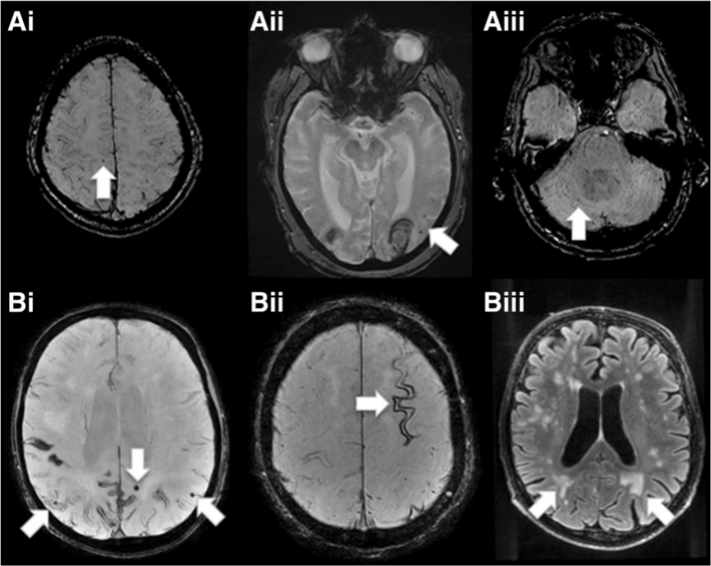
Examples of typical brain magnetic resonance imaging changes associated with vascular cognitive impairment. *A Hypertensive cerebral microbleeds*. Typical appearance of hypertensive cerebral microbleeds in patients around 70 years of age. Note the random distribution including supratentorial superficial white matter (Ai), intraparenchymal (Aii), and infratentorial region (Aiii). *B Cerebral amyliod angiopathy*. Typical manifestaion of celebral amyloid angiopathy in a 72 years old patient, including multiple microbleeds with a labor distribution sparing the deep grey matter and infratentorial region (Bi), superficial siderosis of the covexity (Bii) and periventricular leucoencephalopathy (Biii)
